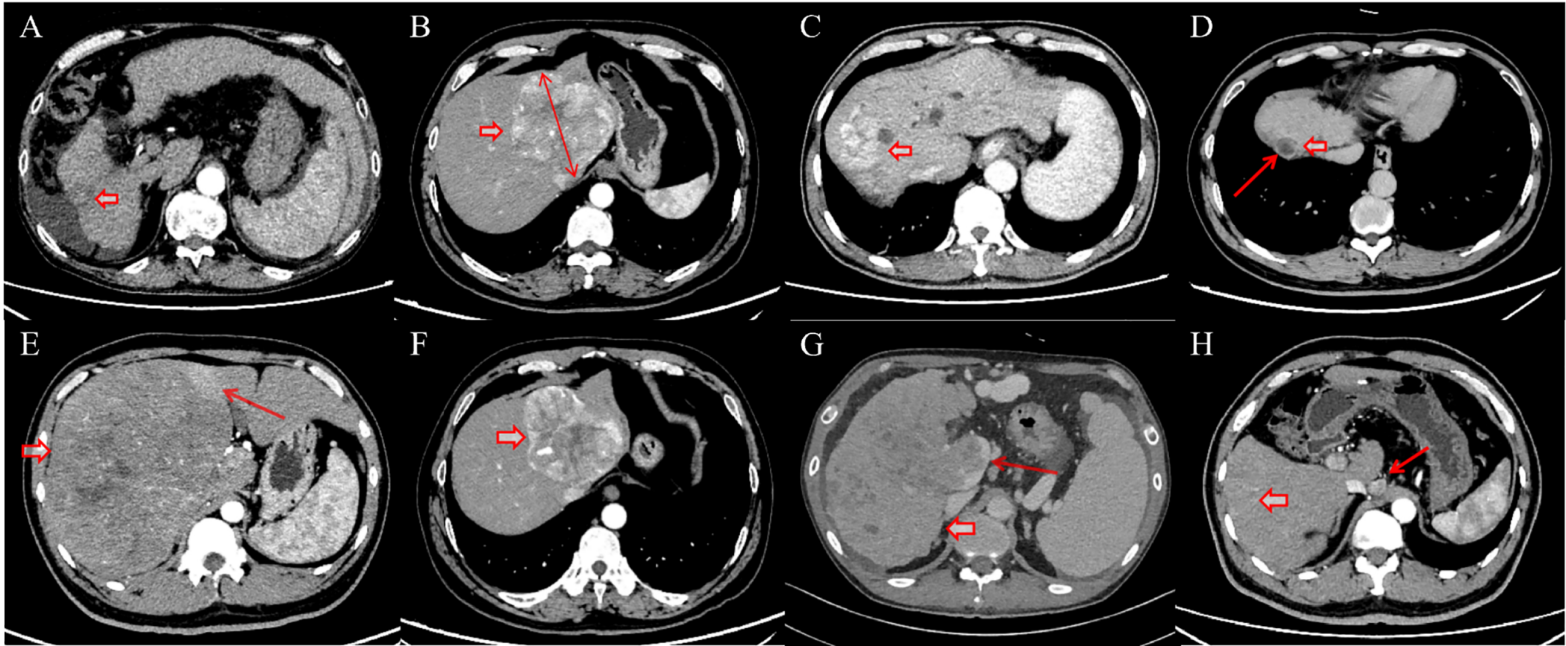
Representative CT images depicting assessment of conventional imaging features in hepatocellular carcinoma (HCC). Red short arrows: HCC. (A) Ascites and liver cirrhosis; (B) Measurement of maximum tumor diameter (without capsule); (C) Irregular tumor margin; (D) Tumor capsule enhancement in the delayed phase with smooth margin; (E) Arterial peritumoral enhancement; (F) Intratumoral arteries and intratumoral necrosis; (G) Portal vein tumor thrombus in the main trunk and right branch; (H) Enlarged retroperitoneal lymph nodes with heterogeneous enhancement.
